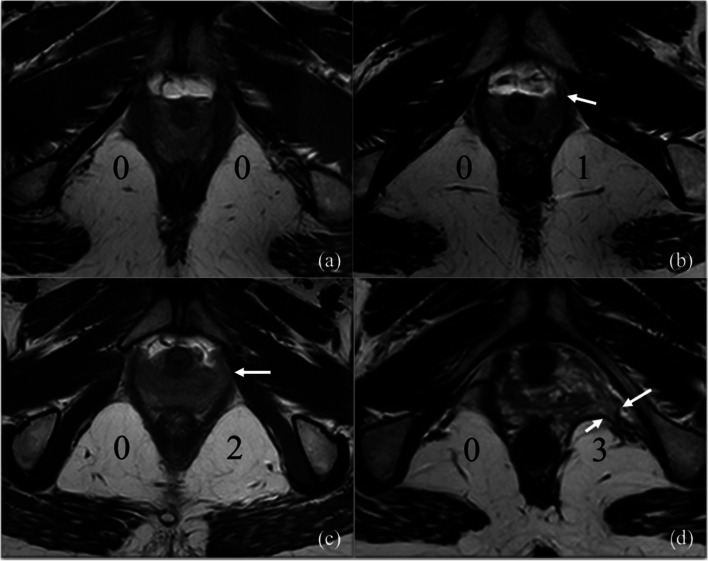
Axial T2-weighted images were performed to assess levator ani injury. The diagnosis of abnormal muscle was based on muscle swelling and defect. a 0 = normal, b 1 = less than half abnormality of the muscle, c 2 = more than half abnormality of the muscle, d 3 = total or near-total loss of the muscle
